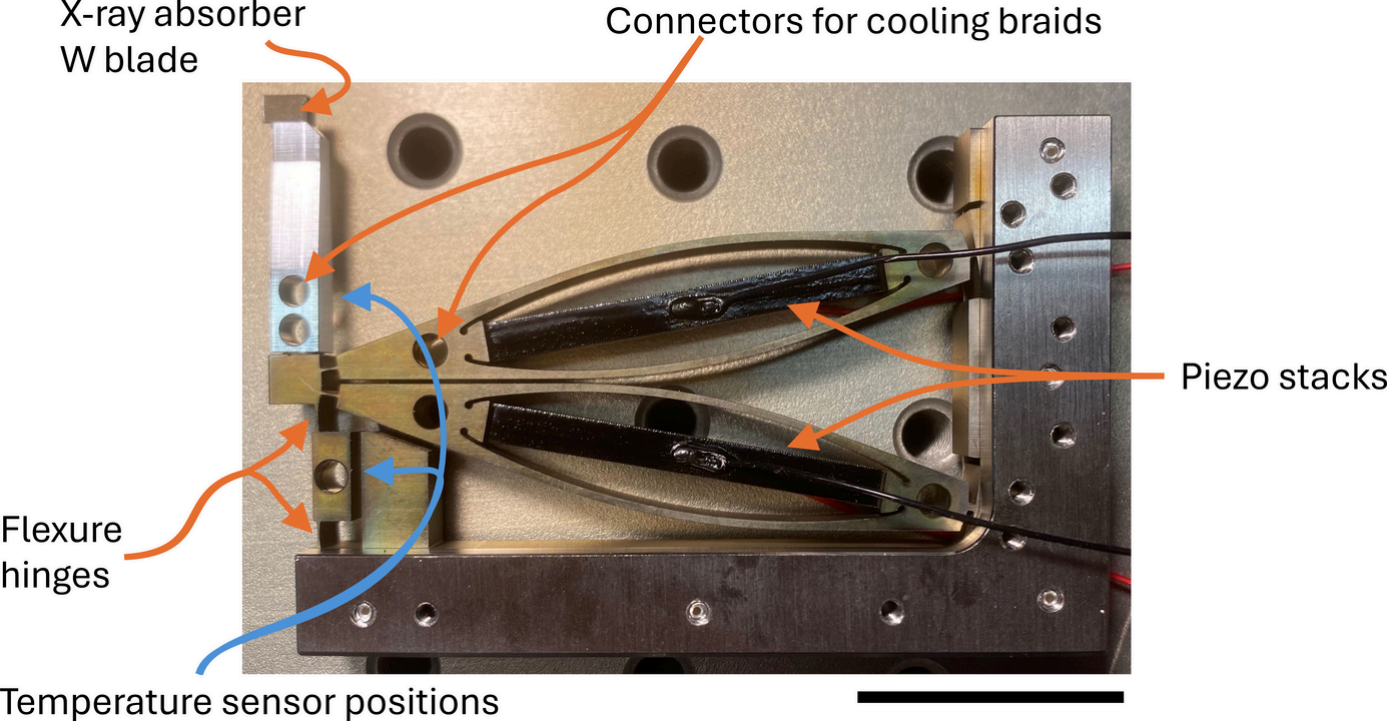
A half shutter module. The X-rays will be absorbed at the tip of the lever structure on the left. The lever is connected via two flexure hinges to the piezo actuators, which operate in inverse directions. An expansion of the upper piezo and a contraction of the lower piezo will result in a movement of the lever to the left, which corresponds to a closing motion. Two such modules are combined for a full shutter. Scale bar: 25 mm.
